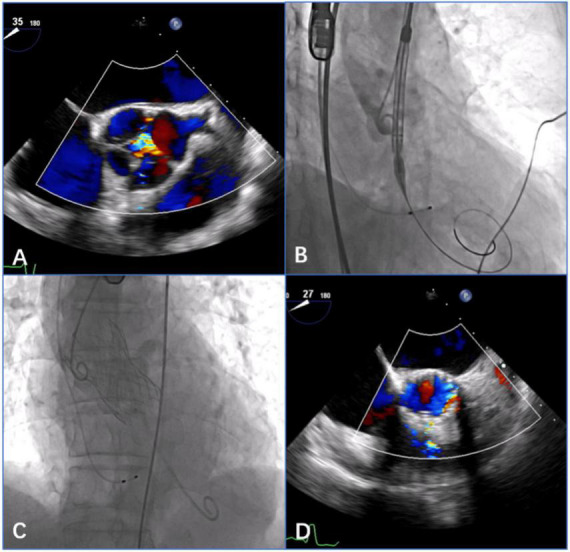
Procedural details of transcatheter aortic valve replacement (TAVR) with the self-expandable Venus-A prosthesis in a patient with quadricuspid aortic valve with aortic stenosis (AS) and regurgitation. (A) 2-Dimensional transesophageal echocardiography (TEE) with color Doppler showed aortic regurgitation in the quadricuspid aortic valve before the procedure. (B) Angiography showed a satisfactory position of the THV prosthesis before deployment. (C) Angiography showed the final position of a 32 mm Venus-A prosthesis without a paravalvular leak after deployment. (D) 2-Dimensional TEE with color Doppler showed no aortic regurgitation postprocedure.
